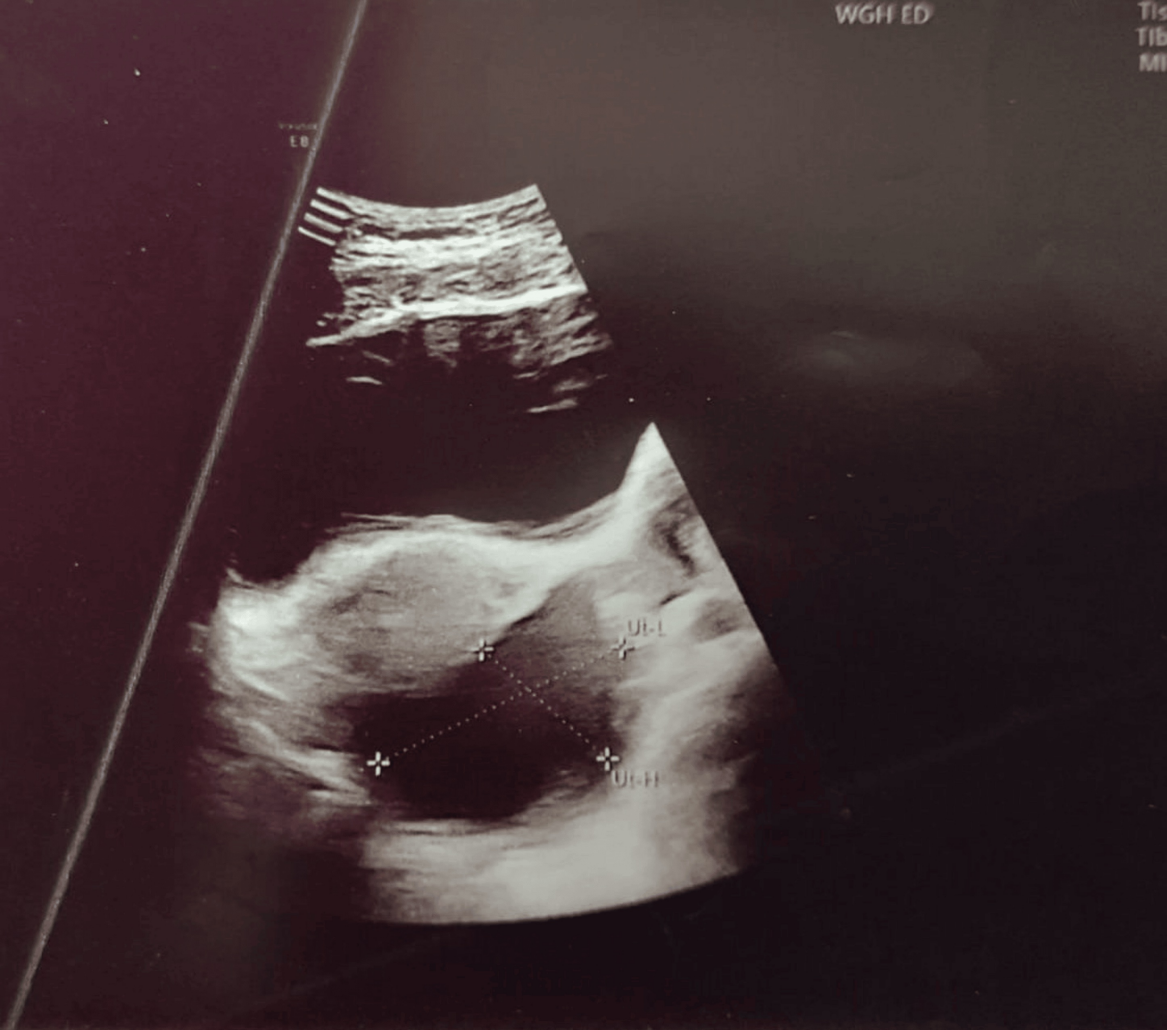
Transvaginal ultrasound (longitudinal view) showing an unilocular cystic lesion adjacent to the normal right ovary This image shows a cystic structure separate from the ovary, with a thin, smooth wall and no internal septations or solid components.
